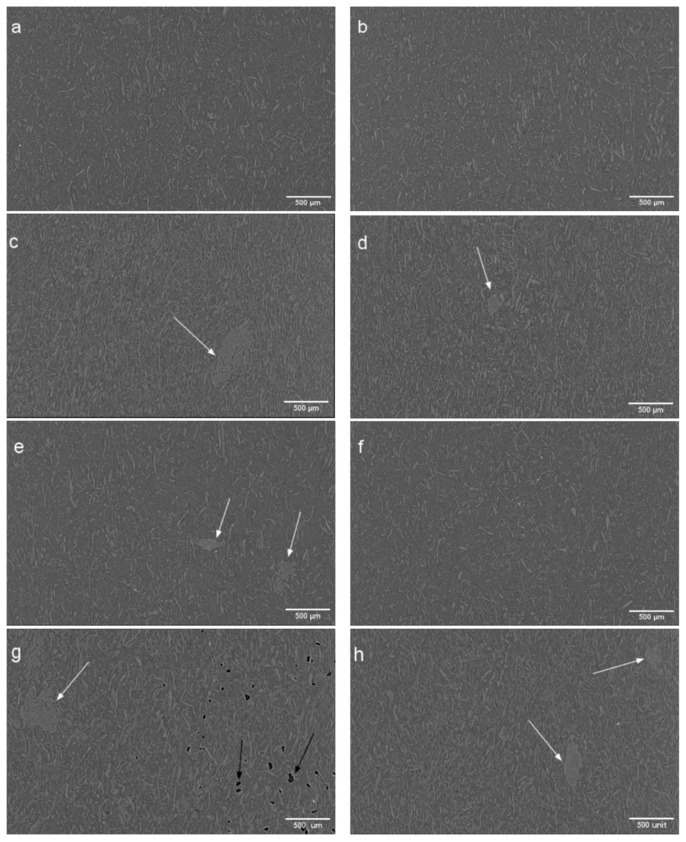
The X-ray tomography images of PLA 15B (a), rPLA 15B (b), PLA 30B (c), rPLA 30B (d), PLA 15UB (e), rPLA 15UB (f), PLA 30UB (g), and rPLA 30UB (h); white arrows indicate the fibre agglomerates, and black arrows show voids.
